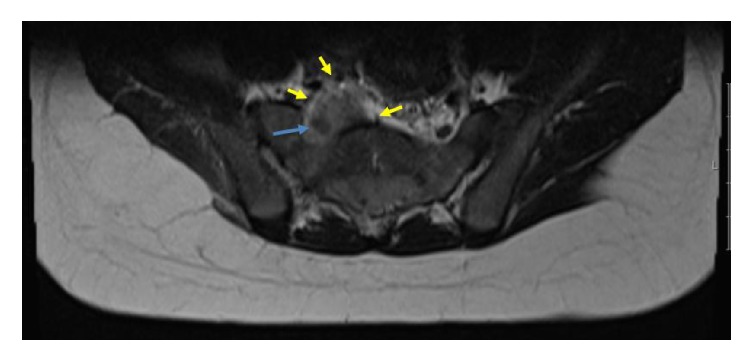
Axial T2-weighted MRI reveals anterior mass extension into the paraspinous soft tissues (yellow arrows), involving the sciatic nerve (blue arrow), which is thickened.
